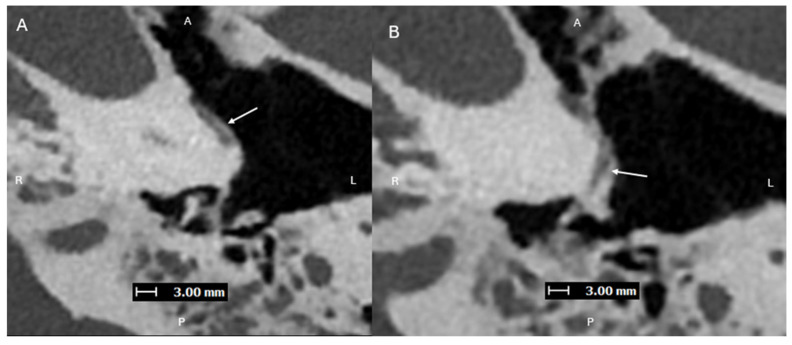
Axial images (superior to inferior (A,B), with A, P, L, R for orientation donating anterior, posterior, left, and right respectively) from a PCD-CT (Naeotom Alpha; Siemens Healthineers, Forchheim, Germany) temporal bone study—with UHR mode (Collimation 120 × 0.2 mm), Tube voltage (120 kVp), slice thickness/increment (0.2/0.1 mm), and sharp Kernel (Hr84) with Quantum Iterative Reconstruction (3)—demonstrating a normal left inferior tympanic canaliculus ITC (solid arrows) passing between the carotid canal and jugular foramen, traveling along the cochlear promontory. The normally small inferior tympanic artery extends through the ITC and is a branch of the external carotid artery, usually the ascending pharyngeal artery. Normally, the ITC, as in this case, is a thin corticated channel that transmits the tympanic branch of the glossopharyngeal nerve (Jacobson’s nerve) and the inferior tympanic artery. The latter is hard to visualize on EID-CT due to its small size. It can enlarge in case of congenital involution of the cervical portion of the internal carotid artery (ICA), forming the aberrant ICA [1,2].
